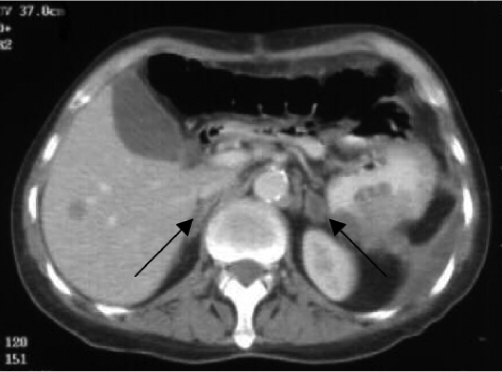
Repeat CT scan of thorax, abdomen and pelvis, five weeks after first scan. Arrows demonstrate interval liquefaction of the adrenal gland haemorrhages.
